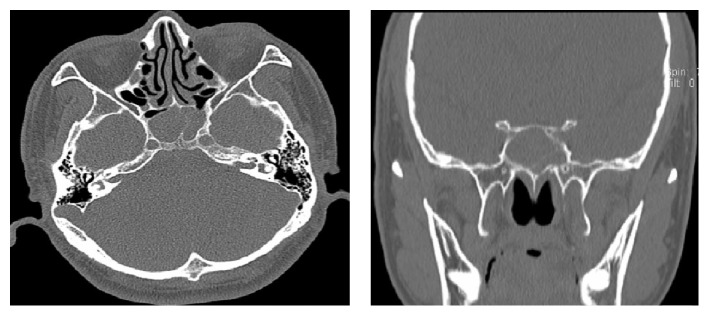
CT scan showed soft-tissue density neoplasms filling with sphenoidal sinus.
